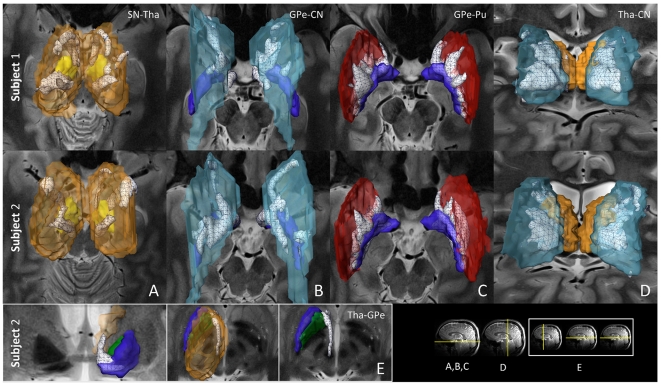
Reconstruction of fiber pathways of the basal ganglia and thalamus. White matter pathways identified using diffusion MRI probabilistic tractography. The white wireframe volumes represent white matter tracts identified as pathways of interest (see Materials and Methods). From left to right: (Panel A) nigrothalamic pathway, (Panel B) and (Panel C) pallidostriatal pathway, (Panel D) thalamostriatal pathway, (Panel E) thalamopallidal pathway. (Bottom right inset) Yellow lines depict the orientation and location of T2-weighted images in each panel. (Panels A, B, C) Axial images at the level of SN; (Panel D) Coronal image at the level of the posterior Tha; (Panel E, left) Coronal image at the level of the anterior thalamus; (Panel E, middle and right) Axial images at the level of the inferior GP. Color code: Caudate nucleus, CN: light blue; Putamen, Pu: red; External globus pallidus, GPe: dark blue; Internal globus pallidus, GPi: green; Substantia nigra, SN: yellow; Thalamus, Tha: orange.
